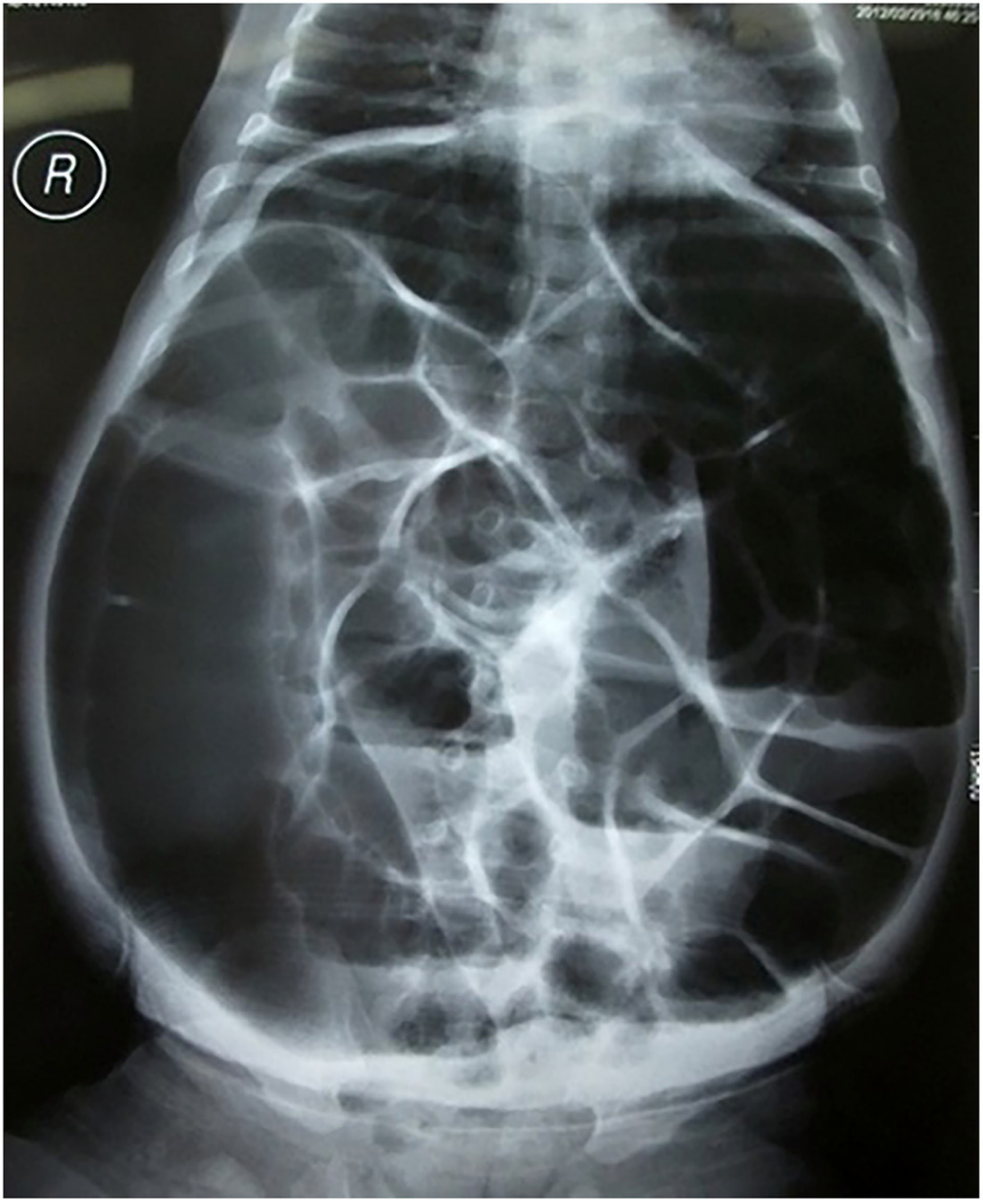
Typical anterior-posterior plain radiographs showed extensive intestinal and colon flatulence and multiple fluid levels.
